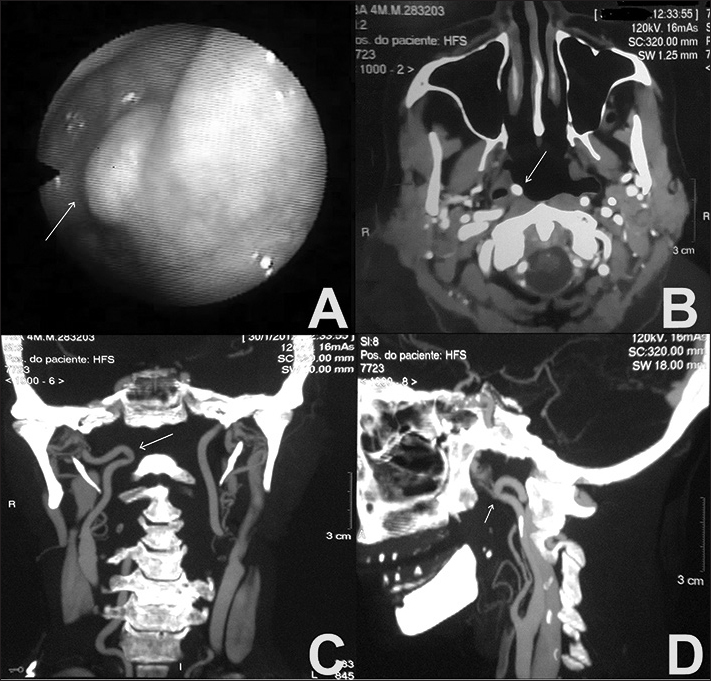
A: Nasal endoscopy image showing a pulsating mass in the lateral recess of the nasopharynx. B, C, D: Contrast-enhanced CT scan of the neck; axial, coronal, and sagittal views, respectively, showing a kinked right internal carotid artery.
